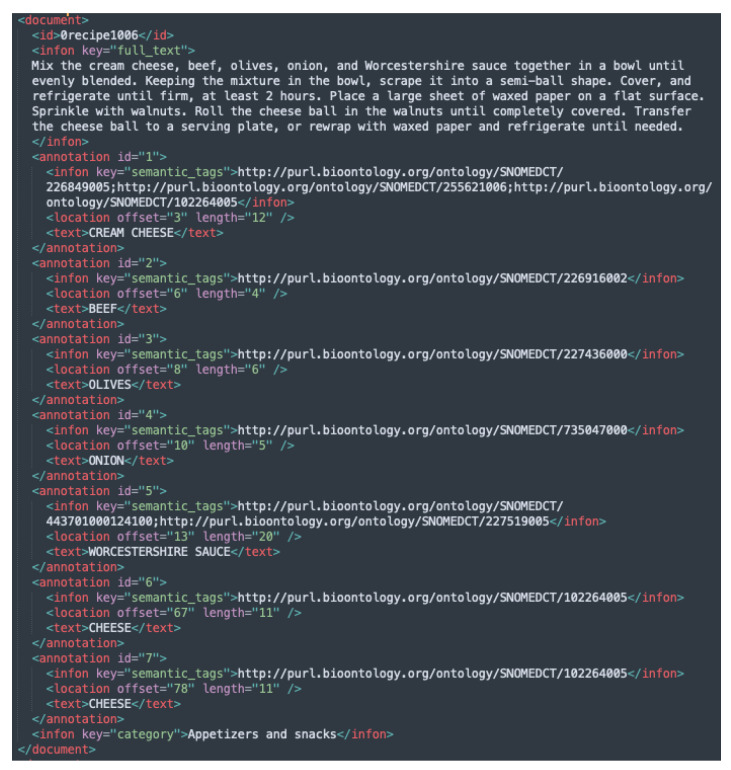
Example of an instance from FoodBase annotated with tags from the SNOMED CT ontology.
